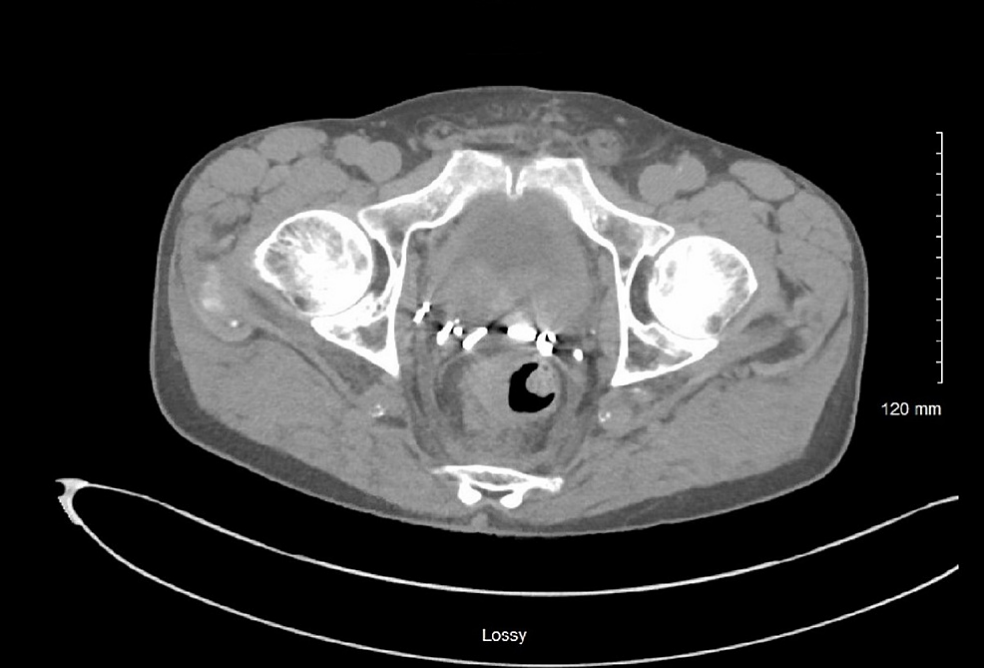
Pelvic CT scan revealing rectal wall thickening.
